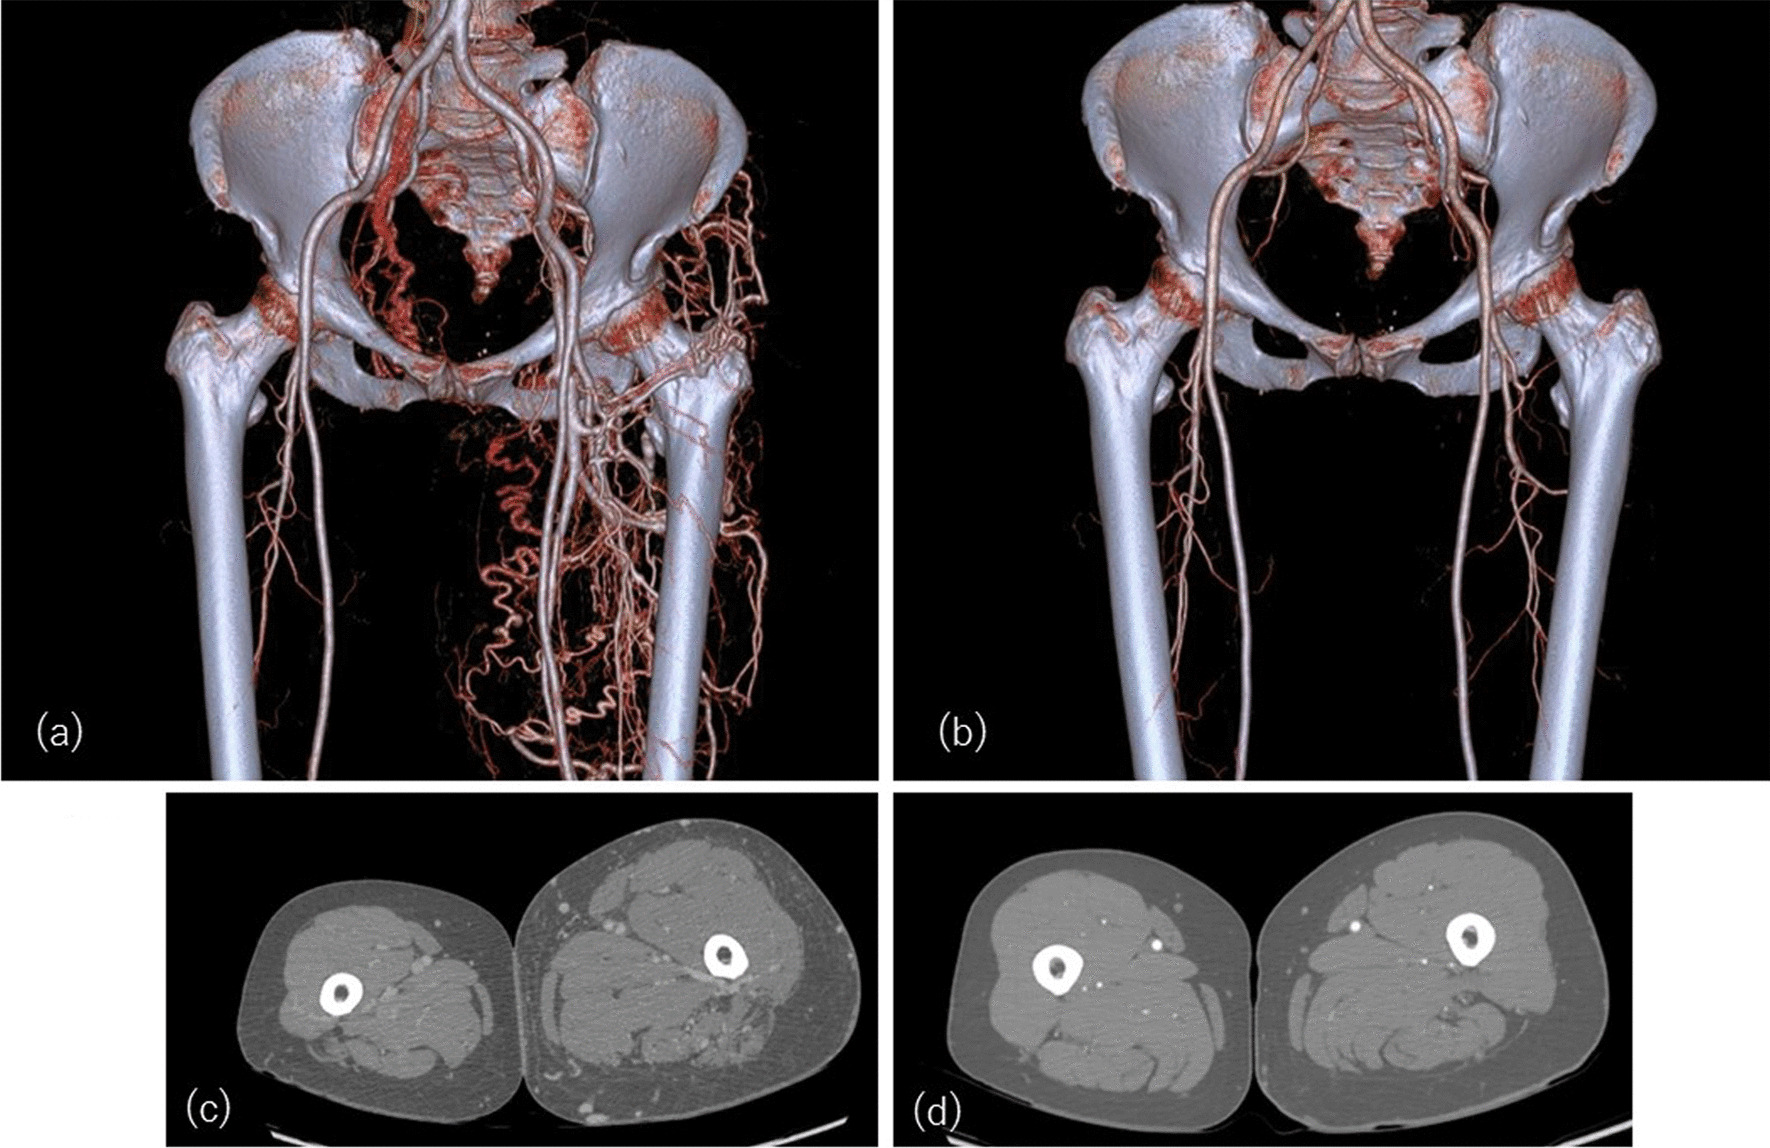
Development of collaterals and arteriovenous fistulas were evident in the left-to-femoral hypogastrium in preprocedure computed tomography angiography (a). Enhanced computed tomography showed significantly reduced collateral veins and arteriovenous fistulas 4 months after the procedure (b). Preprocedure enhanced computed tomography revealed marked arteriovenous fistulas and swelling (c). Postprocedure enhanced computed tomography showed reduced arteriovenous fistulas (d)
